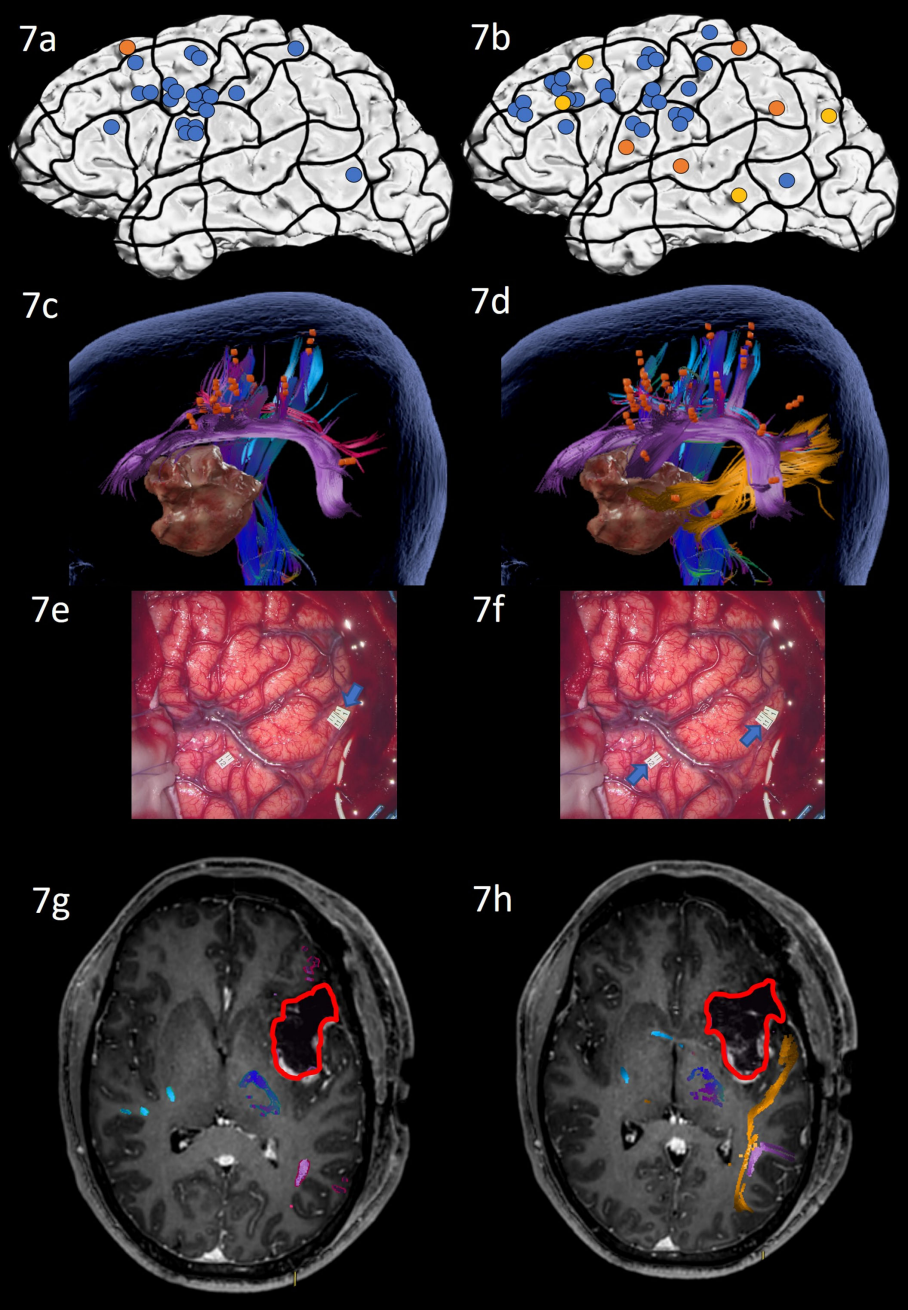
Case 7. Left panels show ON results, right panels AN results. Panels (A, B) nTMS stimulation sites that induced errors; no response and hesitations in orange, semantic errors in yellow and performance errors in blue. Panels (C, D) Fiber tracking results including the lesion, nTMS cortical endpoints in orange and color-coded tracts with CNT in direction-coded, here dark blue, AF in purple, SLF in pink, ILF in yellow, and CF in light blue. Panels (E, F) Screenshot of intraoperative positive cortical sites with tag 1 PoG for ON, and tags 11 + 12 on PoG and the middle STG for AN. Panels (G, H) Overlap of tracking and postoperative T1-weighted imaging after elastic with the resection cavity marked in red.
